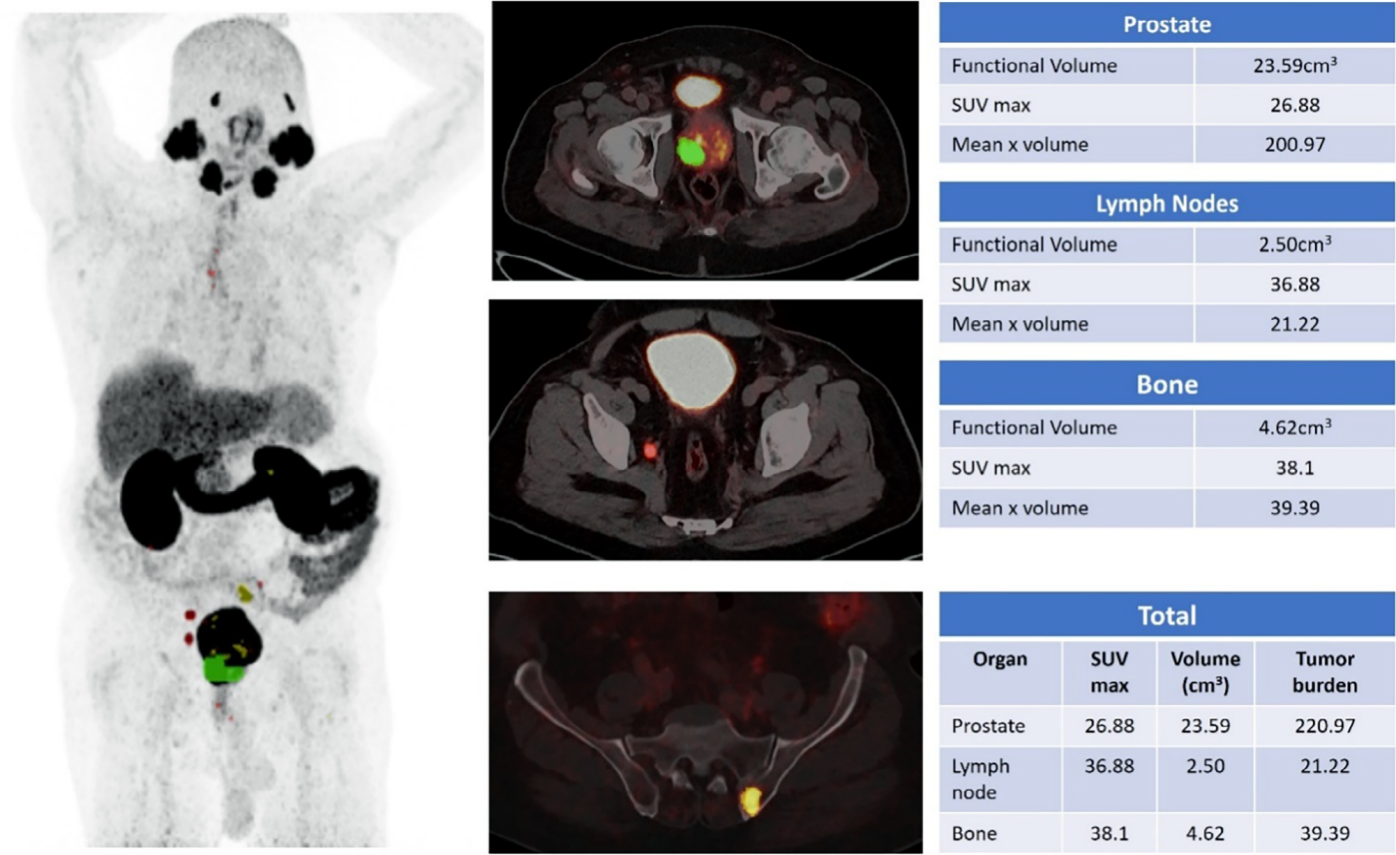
^68^Ga‐PSMA PET‐CT showing prostate lesion at right peripheral zone. Multiple ^68^Ga‐PSMA avid pelvic lymph nodes are observed and left iliac bone is consistent with metastasis.
